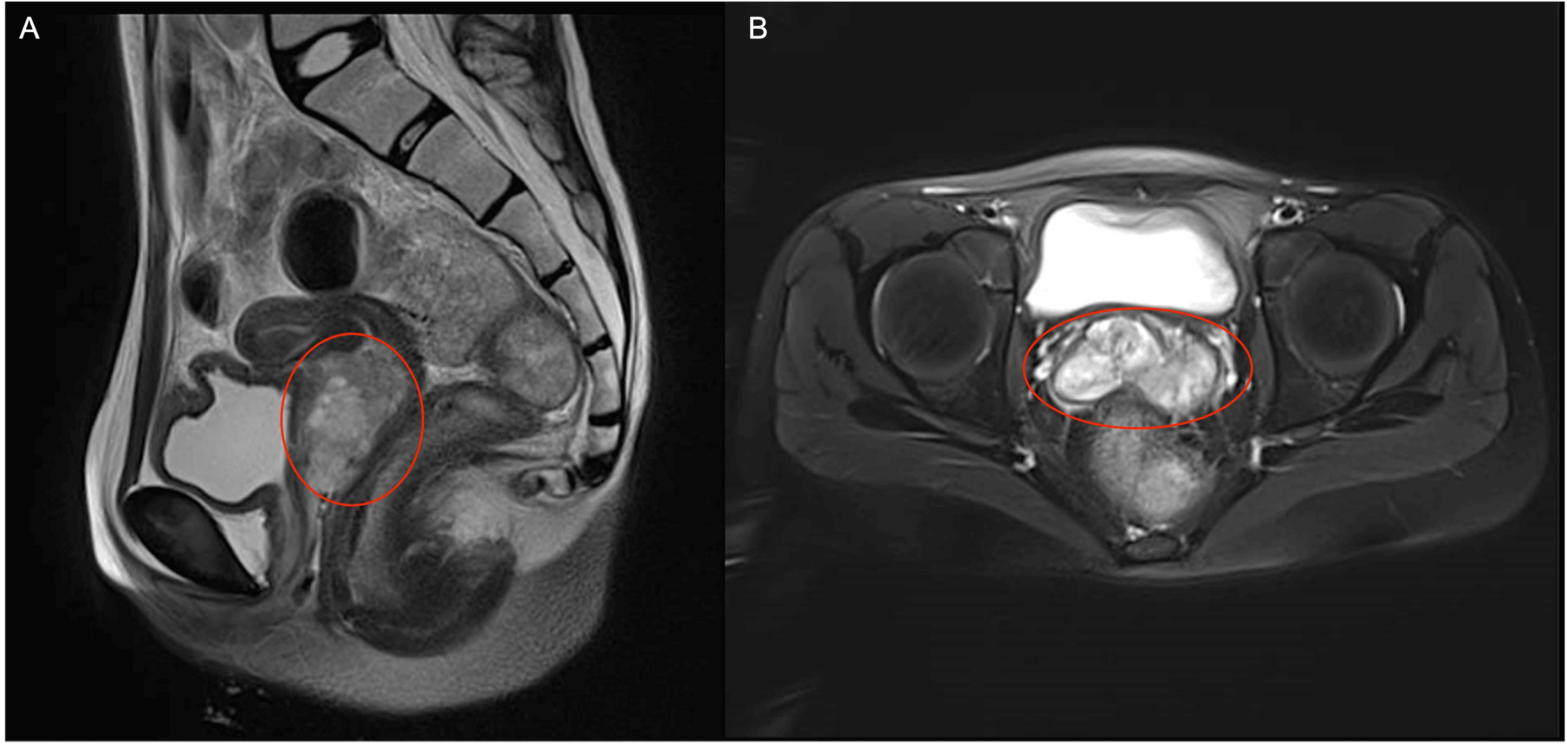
Contrast-enhanced pelvic MRI following cisplatin and EBRT showing the post-treatment residual lesion (red circles) (A) Sagittal view; (B) Axial view EBRT: external beam radiation therapy
